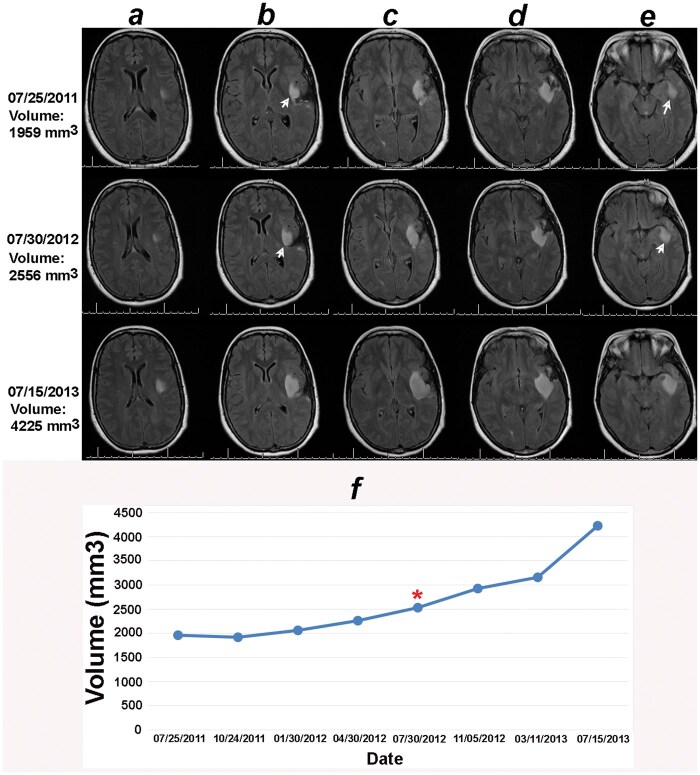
Volumetric analysis combined with the online change-of-point statistical method detects progression on 30 July 2012, 1 year earlier than the date of progression documented in the clinical notes (15 July 2013). Columns A-E correspond to sections within the same MRI series. Notice that though the tumor in the 2D sections containing the largest tumors under columns (C) and (D) did not change between the baseline (25 July 2011) and 30 July 2012, the 2D sections in columns B and E increased in size between the baseline MRI of 07/25/2011 and the date of progression detected by the change-of-point method, 30 July 3012 (white arrows). (F) The online change-of-point analysis detects progression at the red Asterix. The clinical notes detected progression 1 year later.
